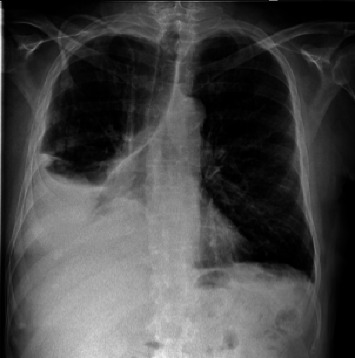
X-ray showing recurrence of hydrothorax.
